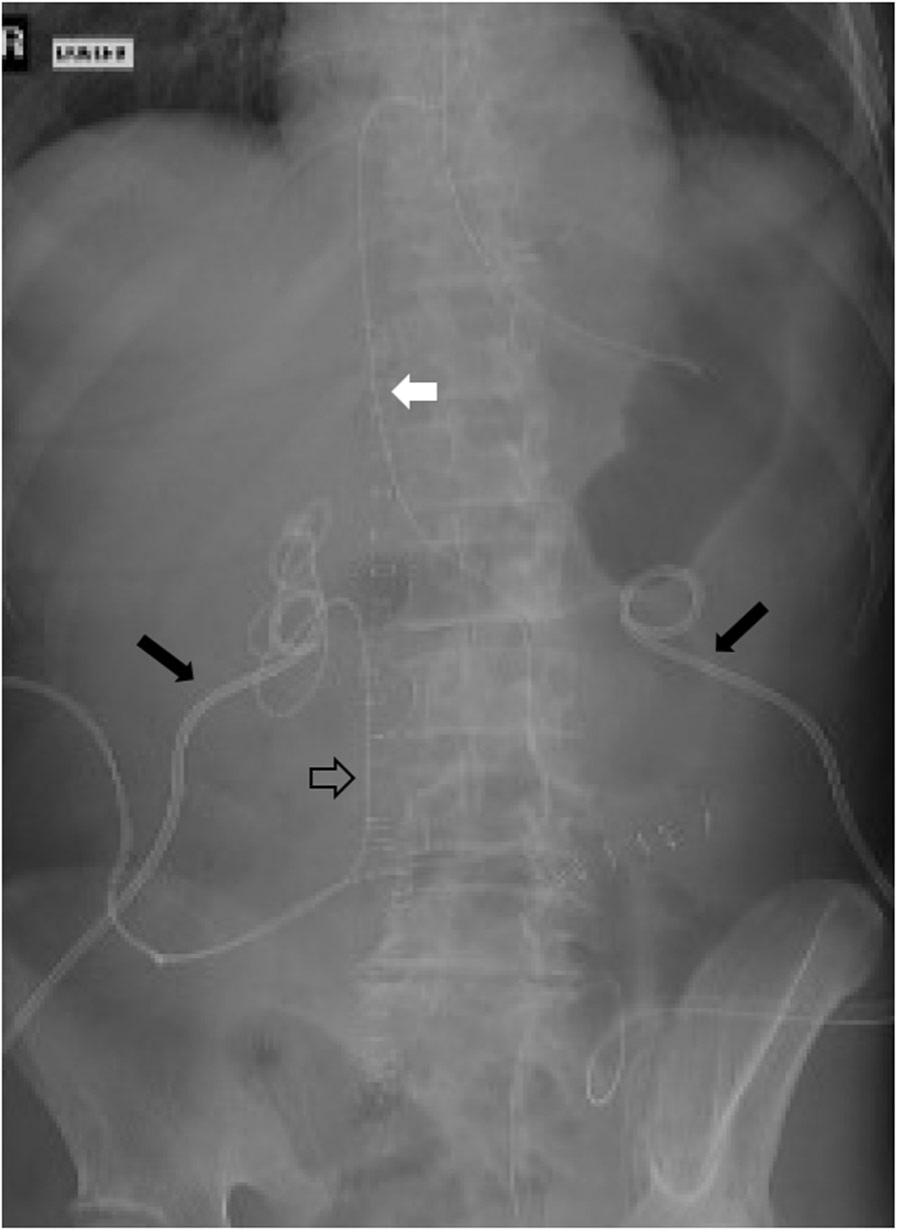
Postoperative X-ray. Black arrows: bilateral nephrostomies. White arrow: left single J stent into the right atrium. Empty arrow: right single J stent into the right kidney
